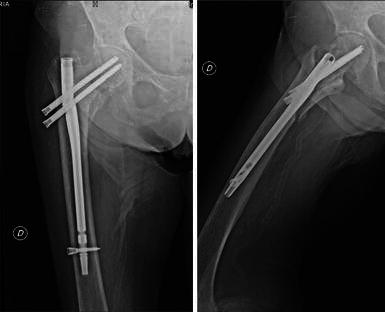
Six-month follow-up radiographs show nonunion of the intertrochanteric fracture and breakage of the nail. Note the sliding of the cephalic screws and the failed sliding of the nail around the distal screw. On the lateral view, the tip of the nail is abutting the anterior cortex
